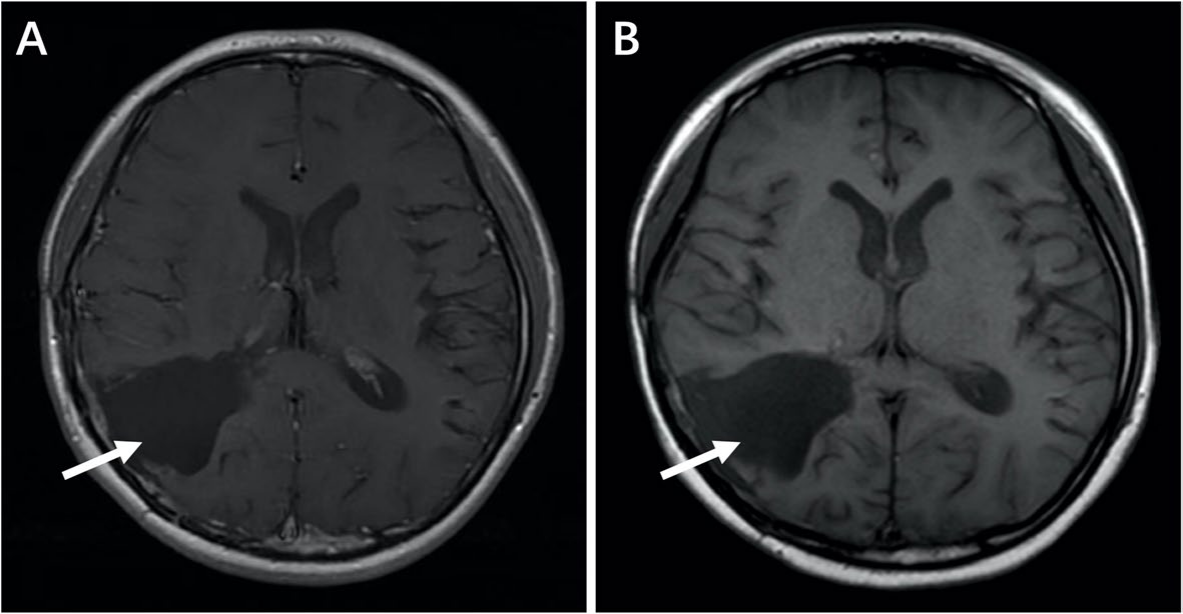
Brain MRI showed no significant change compared with previous (A, B). A 2019.9; B 2020.04
